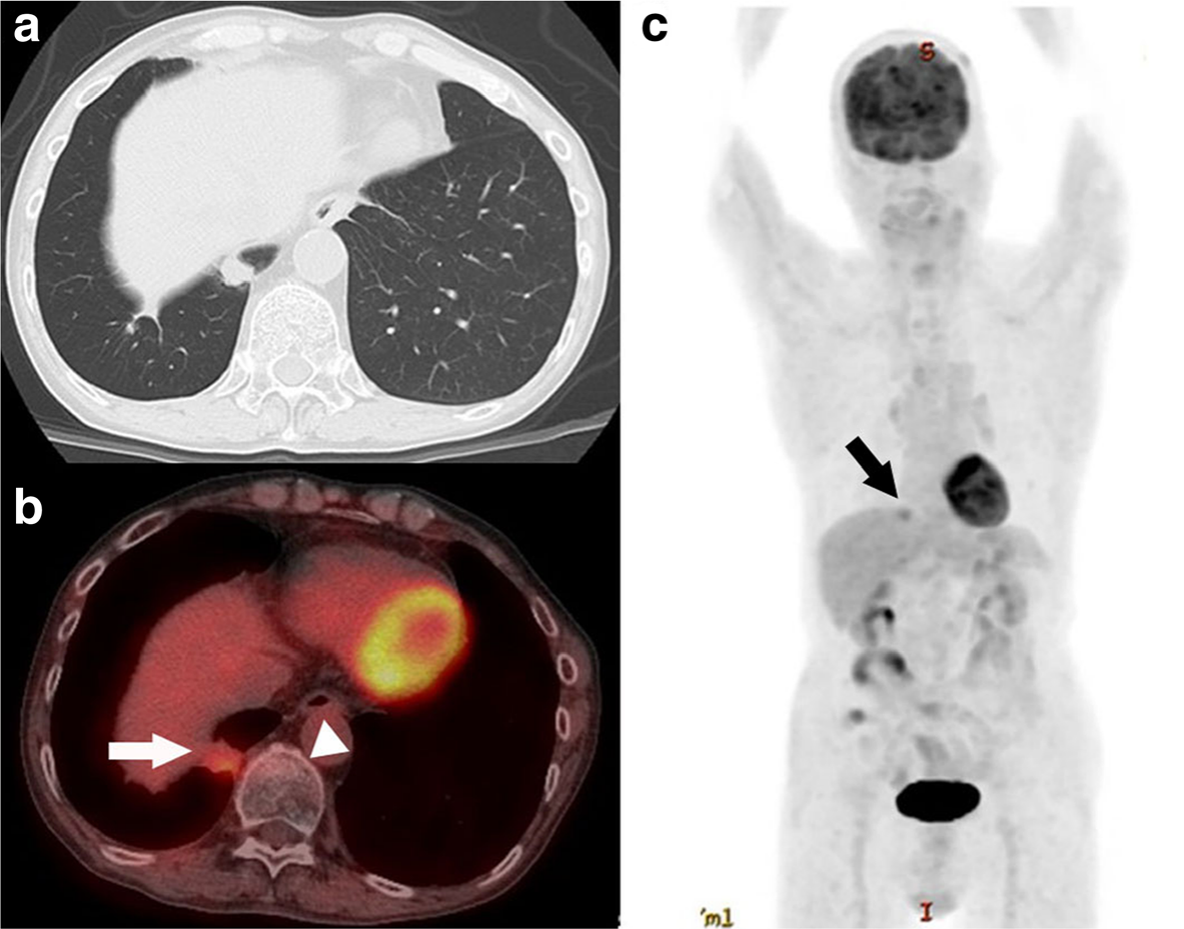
Images of computed tomography (CT) and Positron emission tomography (PET) /CT before admission to the hospital. a CT showing a pulmonary mass in the right lower lobe. b and c: PET/CT showed the pulmonary mass had maximal standardized uptake value (SUVmax) of 3.88 and the lower thoracic esophagus had SUVmax of 2.37
